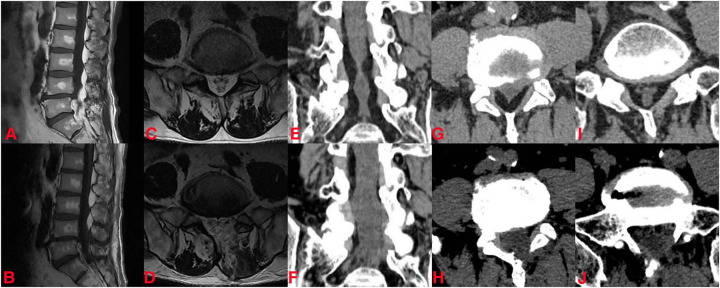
The preoperative and postoperative imaging data of the patient. Preoperative (A) and postoperative (B) sagittal MRI image; preoperative (C) and postoperative (D) axial magnetic resonance imaging (MRI) image at the L5–S1 level; preoperative (E) and postoperative (F) coronal CT; preoperative (G) and postoperative (H) axial CT at L4–5 level; preoperative (I) and postoperative (J) axial CT at the L5–S1 level.
